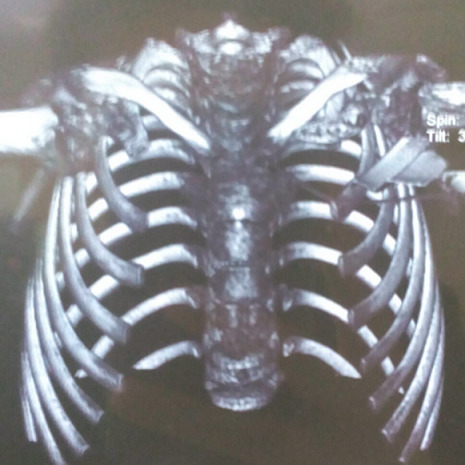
chest CT reconstruction showing the clavicular shortening
